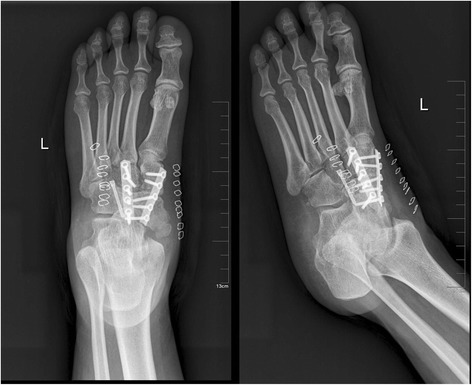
Postoperative radiograph with talonavicular-cuneiform arthrodesis
